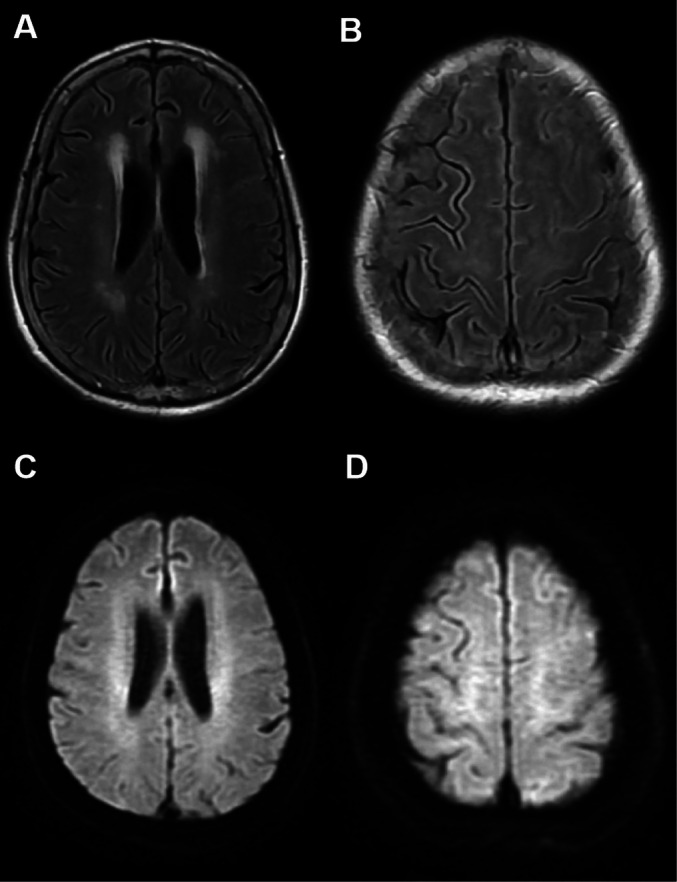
Axial FLAIR MRI sequences (A and B) and DWI (C and D) showing near‐complete resolution of leptomeningeal hyperintensities.
